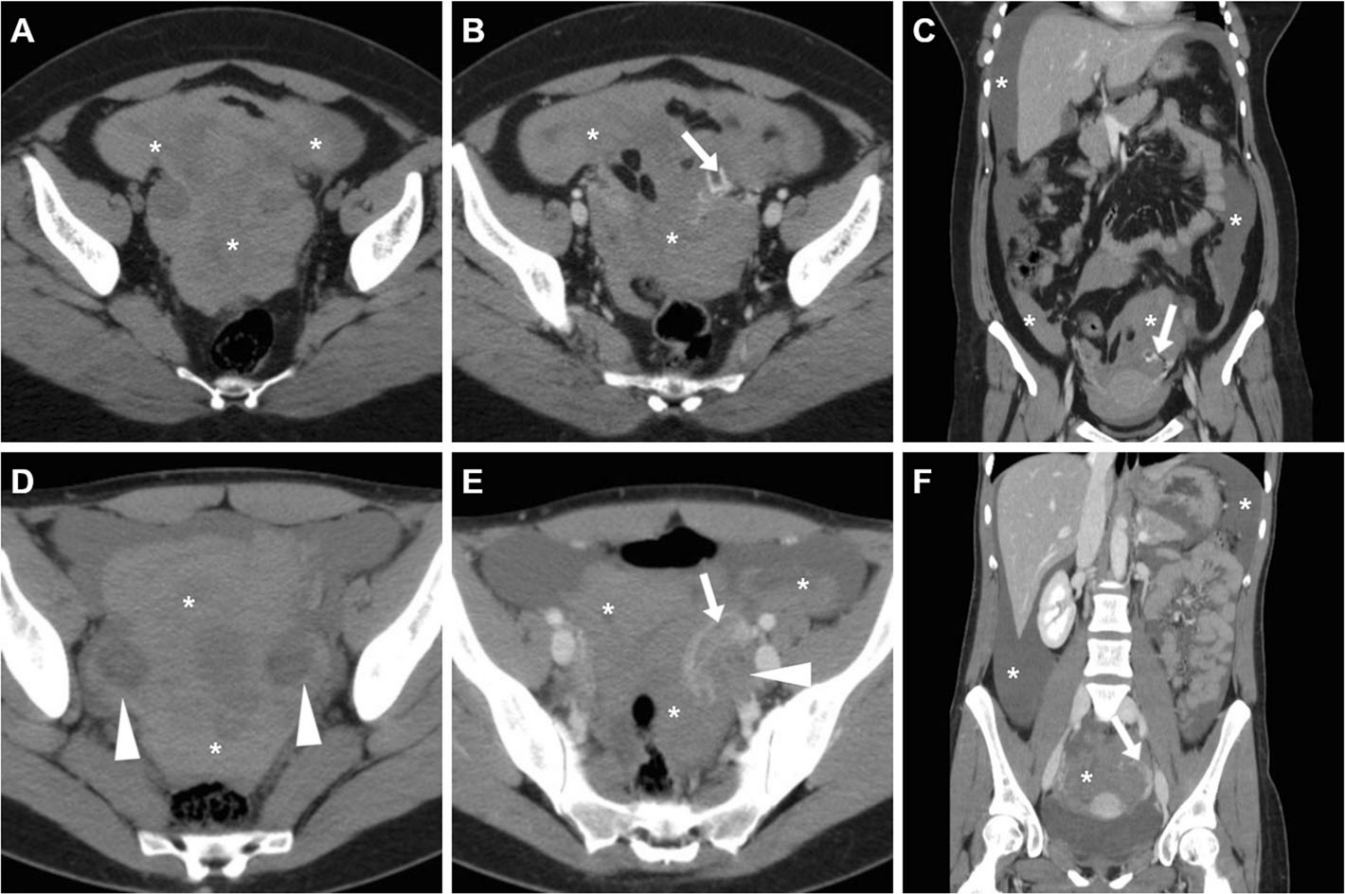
Two surgically proven cases of haemoperitoneum from early extrauterine pregnancy. a–c Unenhanced (a) and postcontrast (b, c) CT images show diffuse hyperattenuating peritoneal effusion (asterisk), enlarged left ovary containing a focal contrast extravasation (arrows in b and c) indicating active bleeding. d–f Unenhanced (d) and postcontrast (e, f) CT images show haemoperitoneum (asterisk), bilateral cystic-like ovarian lesions (arrowheads) with active haemorrhage on the left side (arrows in e and f)
